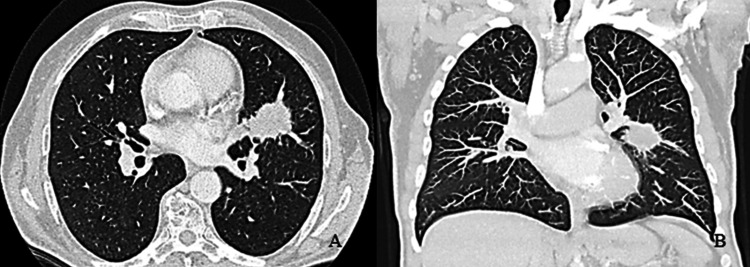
(A) Axial and (B) coronal CT scan (lung window) showing the left upper lobe lesion
